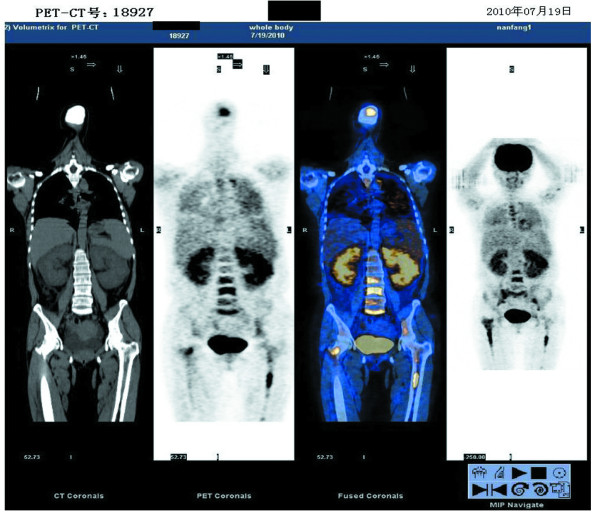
PET-CT shows foci with high signal intensity in bilateral kidneys, multiple vertebra, bilateral sacrum and ilium.
